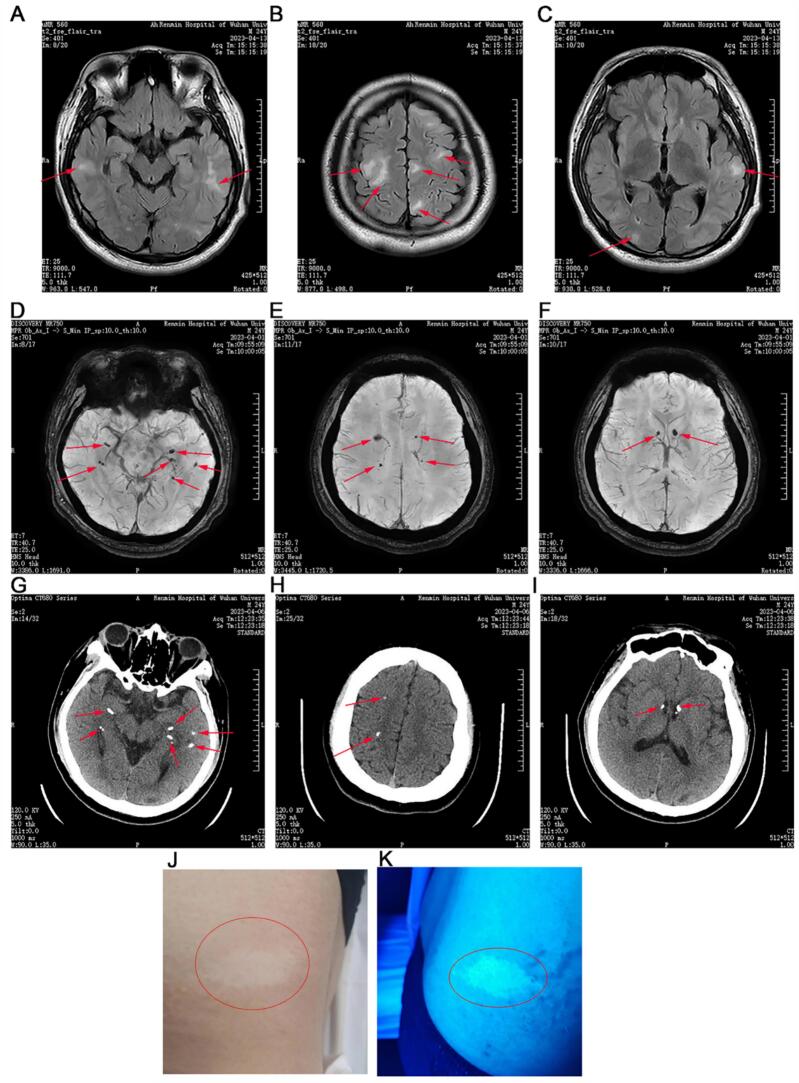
Clinical phenotypic characteristics of the proband. A-C: anti-AQP4 test result. A-C: MRI scaning results. D-F: SWI scanning results. G-I: CT scaning results. J-K: Skin examination results. The red arrow indicates the affected area. The red circle indicates a skin area with depigmentation. (For interpretation of the references to colour in this figure legend, the reader is referred to the web version of this article.)
